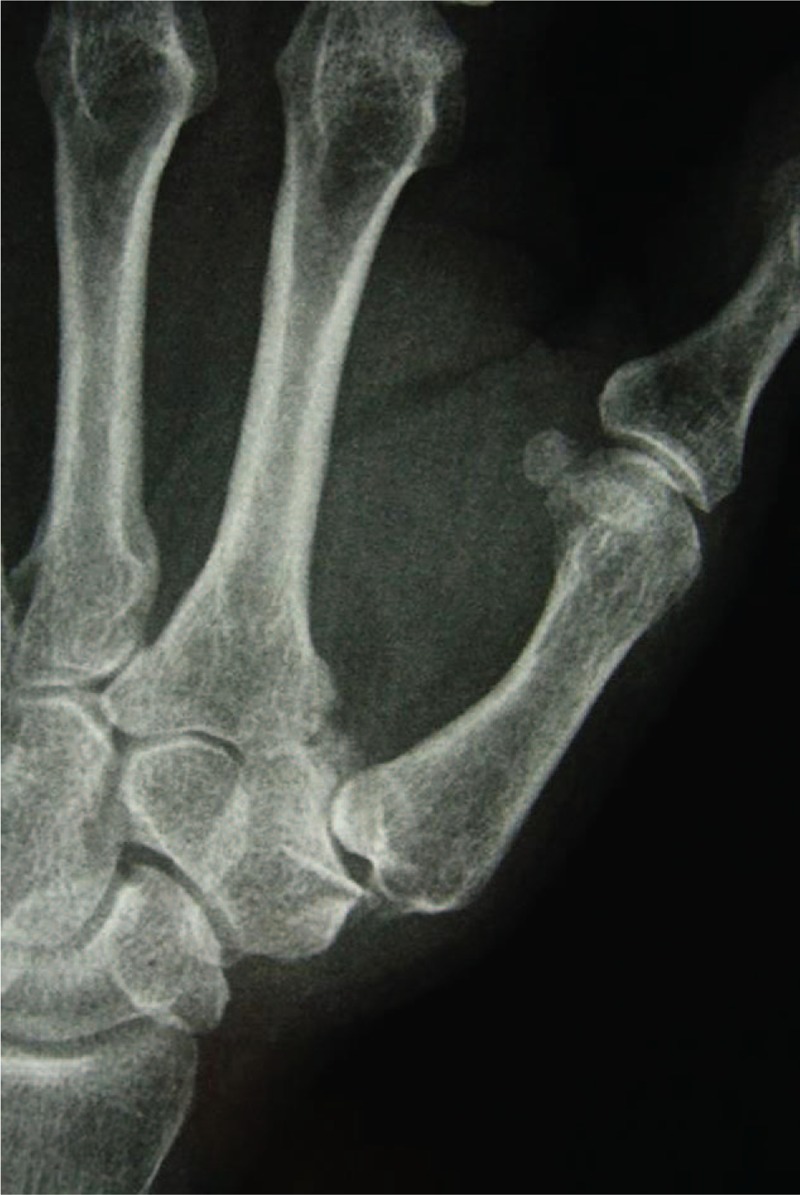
Hand x-ray showing the height of the trapezial space to be10 mm and left thumb CMC arthritis. CMC = carpometacarpal.
